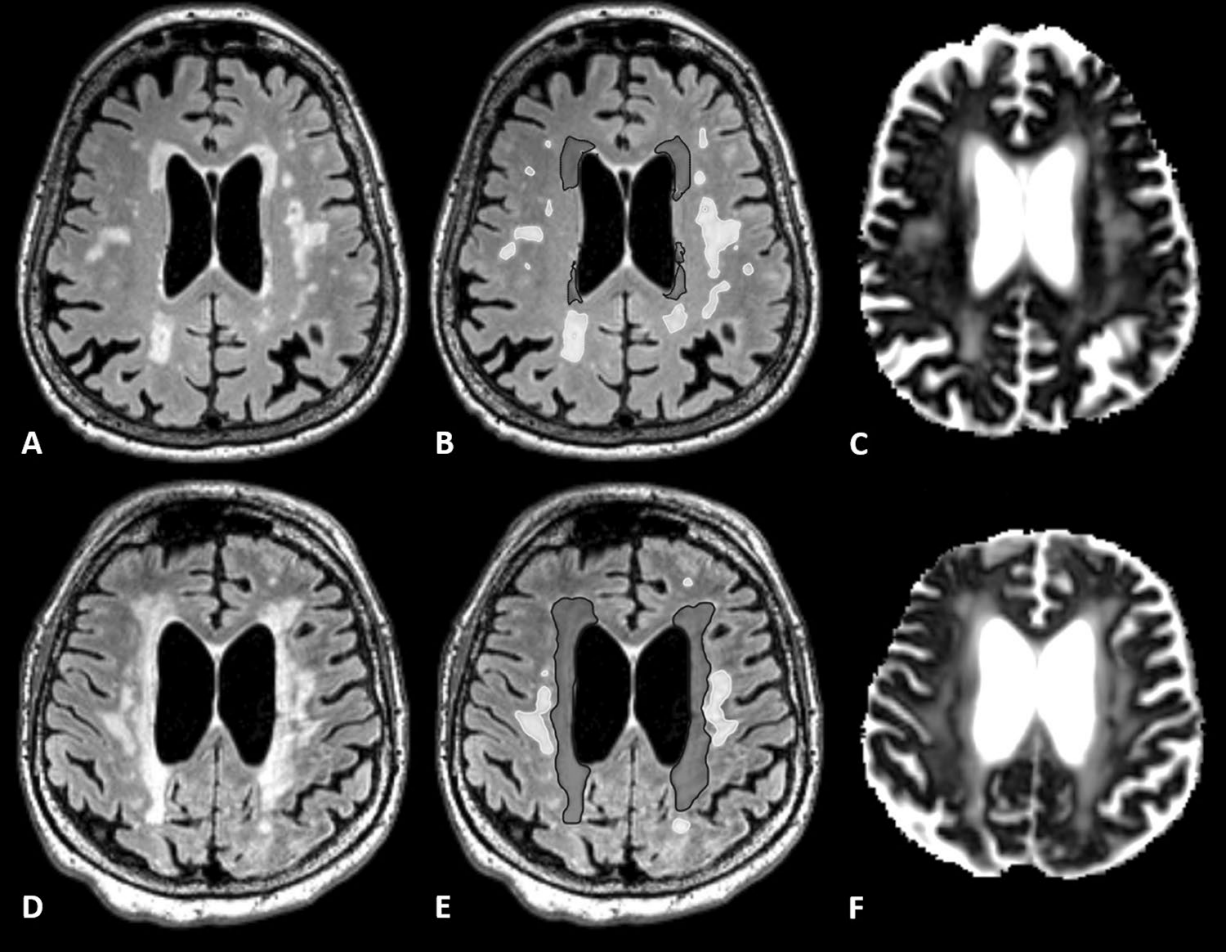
Axial FLAIR reformats in patients without (A) and with imaging features of iNPH (D) with corresponding superimposed segmentations (B, E) divided into periventricular hyperintensities (dark grey) and deep white matter lesions (light grey). Related parametric V-CSF-maps are shown in (C) and (F).
